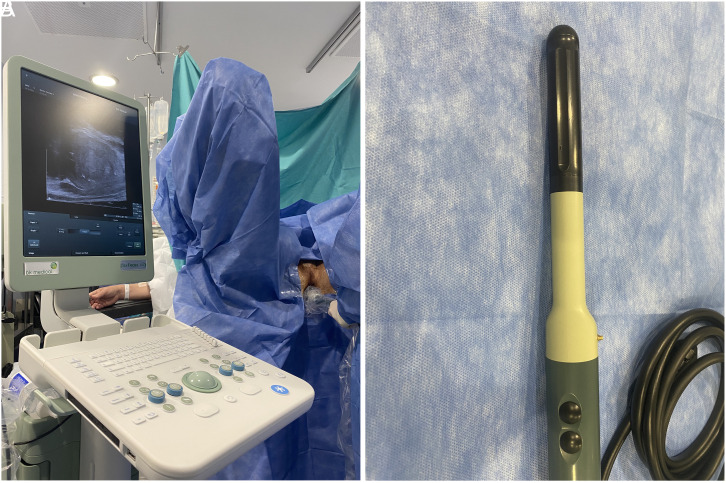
A, B. (A) High-resolution ultrasound machine (5000 BK, Cambridge MA, England), (B) in combination with an endocavitary transducer.
